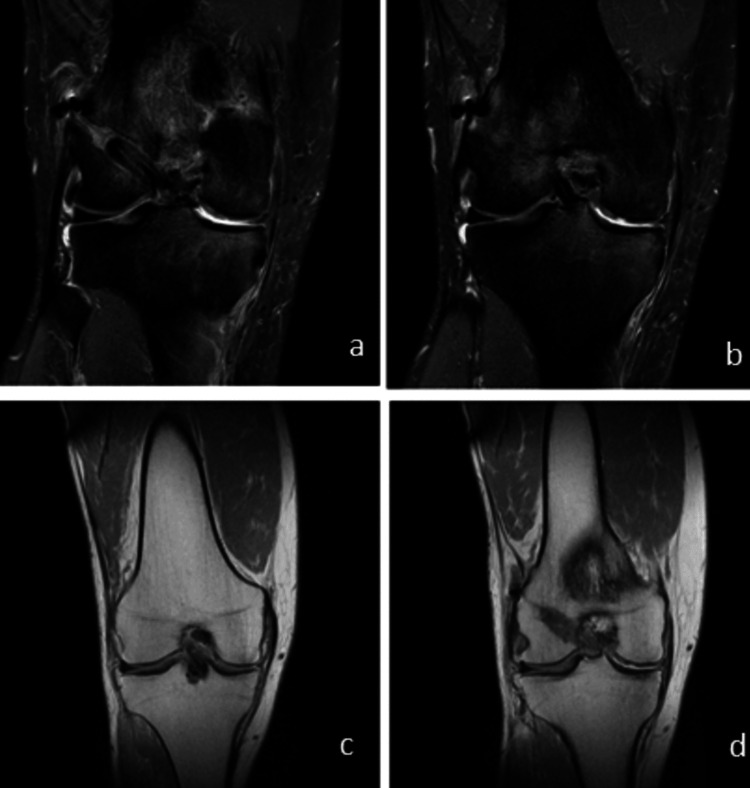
A T2-weighted image of a patient at one-year follow-up. MRI coronal image showing integration of the graft in the femoral tunnel (a) and healthy ligament (b). MRI coronal image showing healthy ACL (c) and graft integration (d).
